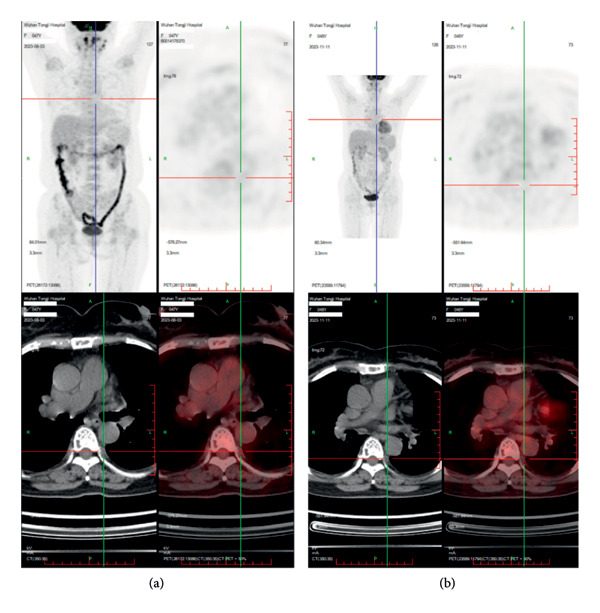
Comparison of extramedullary lesions on PET‐CT in the patient before and after treatment. Prior to GPRC5D‐CAR‐T therapy, the patient exhibited a soft‐tissue density lesion measuring approximately 12 mm × 4 mm adjacent to the thoracic vertebrae (a), which was suggestive of an extramedullary lesion. However, 3 months following treatment, the soft tissue density lesion had completely resolved (b).
